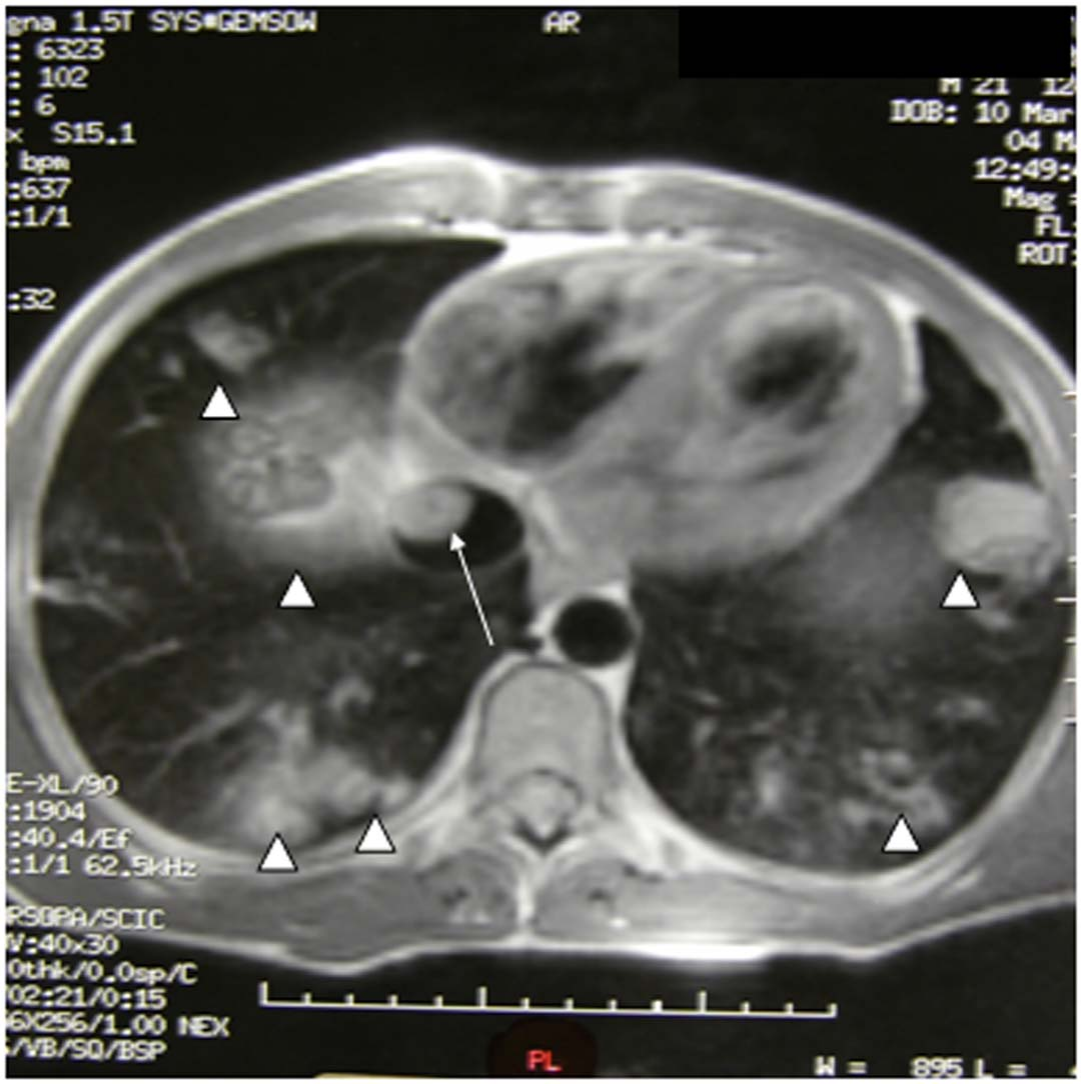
Computed tomography showing IVC cyst (arrow) and multiple CE pulmonary embolisms (arrowheads) (patient 7).
